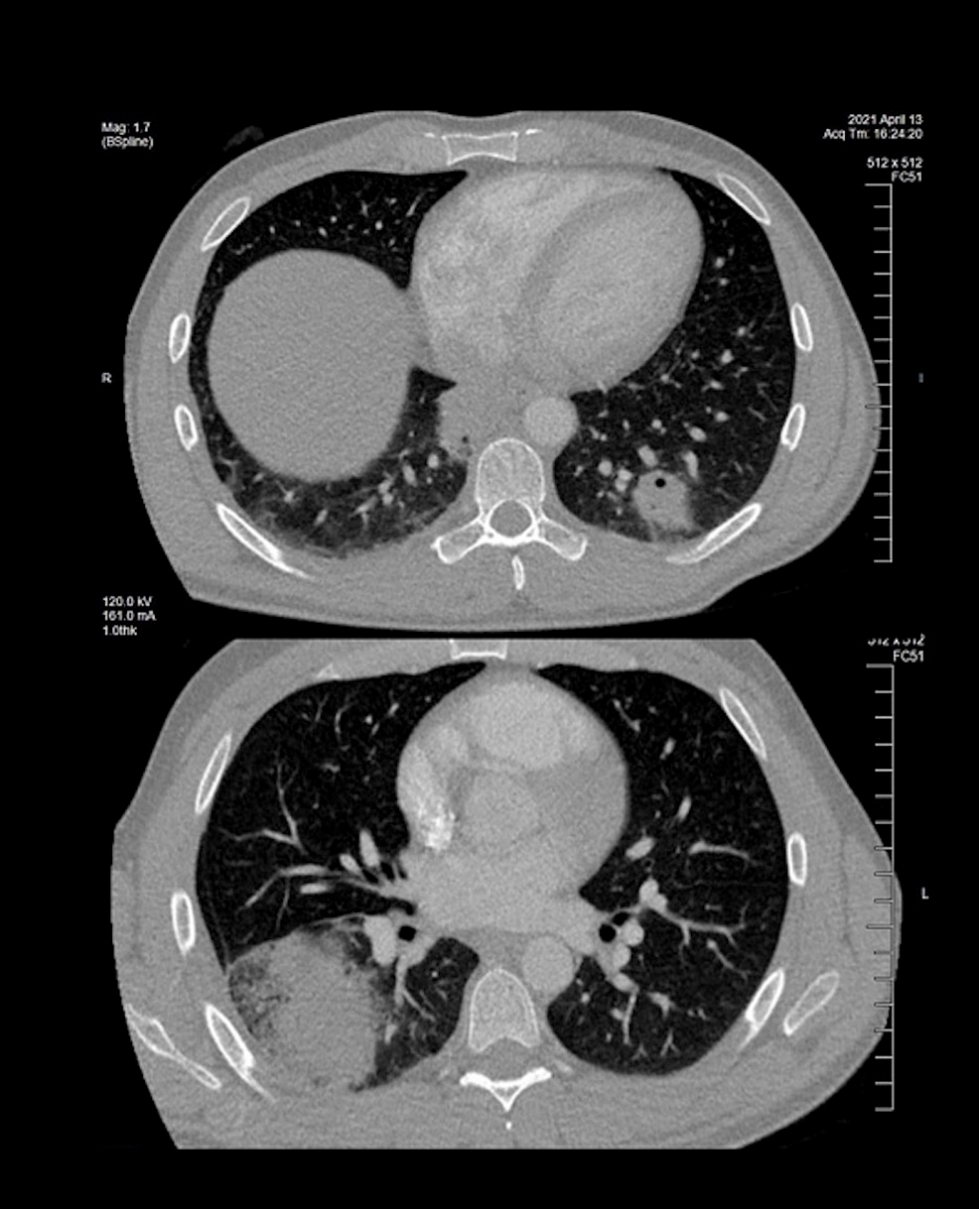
Computed tomographic angiography of the chest (axial view). Axial computed tomographic angiography images of the chest were obtained from the thoracic inlet through the lung bases at 1 mm intervals with IV contrast (75 mL Isovue 370). There were no pulmonary arterial filling defects to suggest acute pulmonary embolus. The bottom image demonstrates a large focus of consolidation and infiltration within the right lower lobe and within the azygous-esophageal recess. The top image shows a 4 cm rounded focal opacity of the left lower lobe with cavitation.
